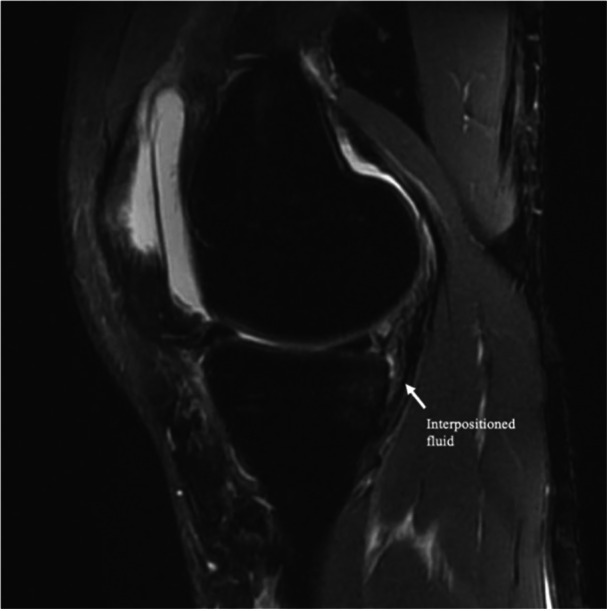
Magnetic resonance imaging (MRI) view: fluid interposition between the tibial edge and semimembranosus tendon.
